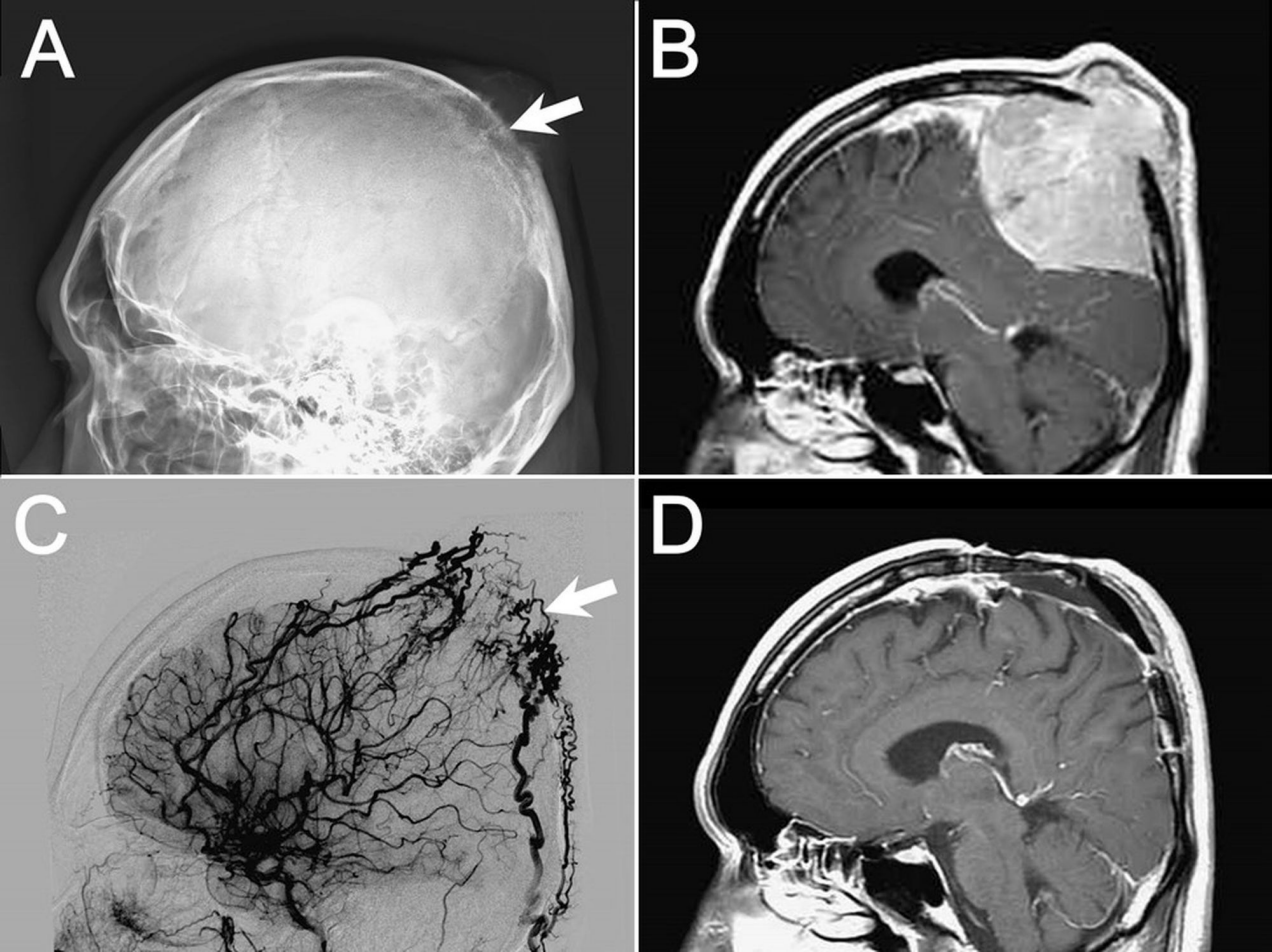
Preoperative and postoperative images. Figure 1A: Skull erosion (arrow) was found in the plain film. Figure 1B: In preoperative magnetic resonance imaging (MRI) (gadolinium-enhanced T1-weighted sagittal view), a large parasagittal tumor was seen with homogenous contrast enhancement and bone erosion, causing both intracranial and extracranial mass effect. Figure 1C: The tumor was highly vascularized (arrow) as demonstrated by angiography. Figure 1D: Postoperative MRI at one year showed no evidence of residual or recurrent tumor.
